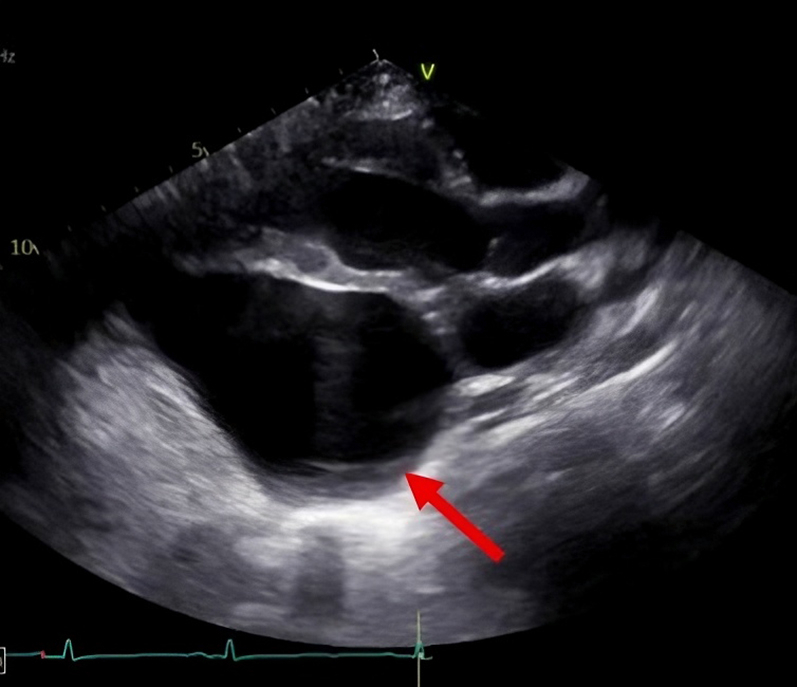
Transthoracic Echocardiogram A large cystic extracardiac mass adjacent to the left ventricular posterior wall (red arrow) in a parasternal long-axis view.
